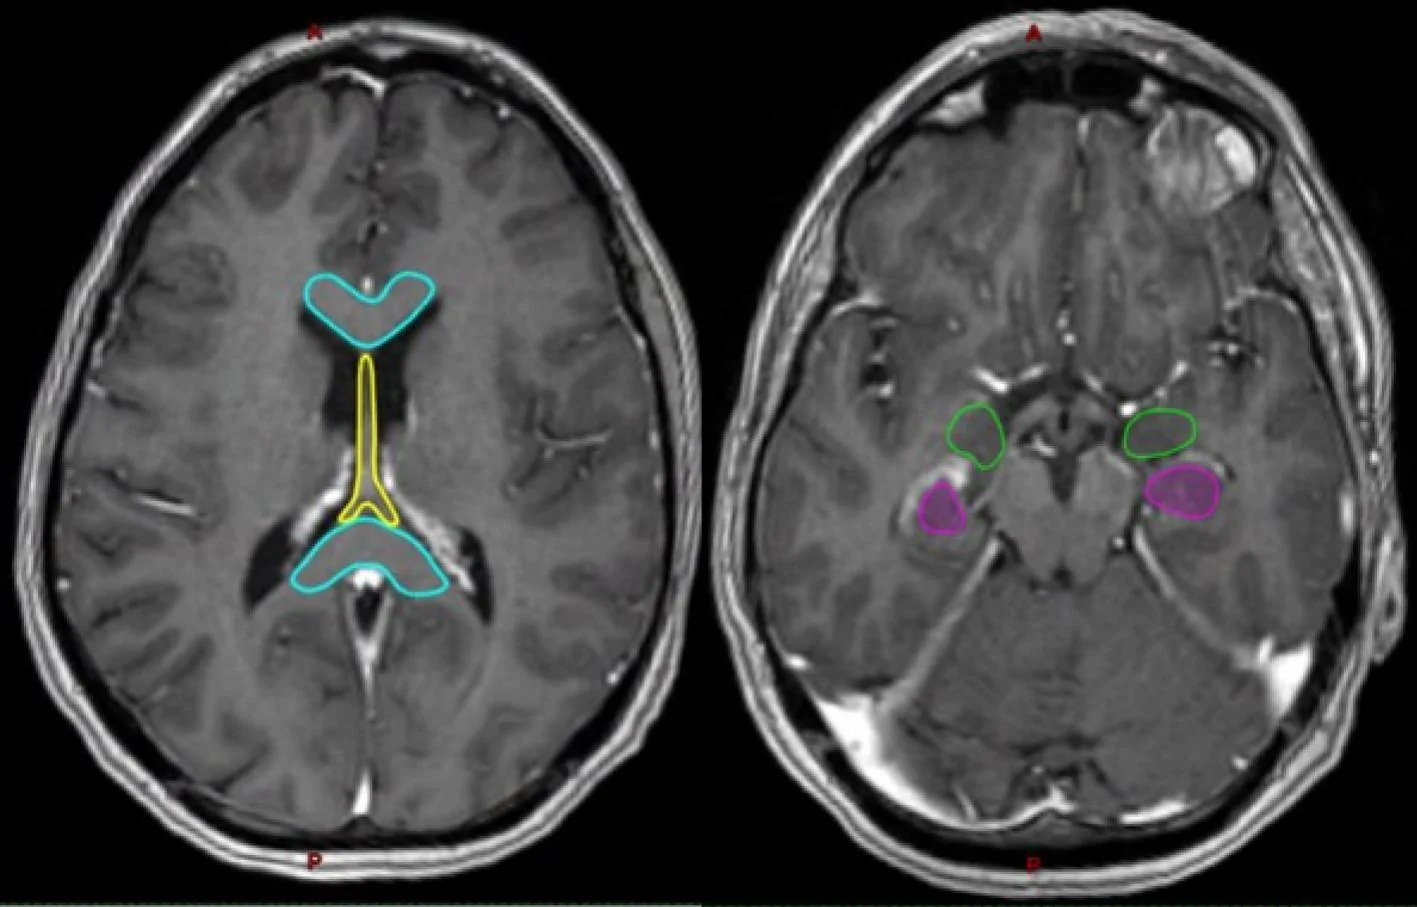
Representative MC contours on MRI brain with contrast which includes corpus callosum (cyan), fornix (yellow), amygdalae (green), and hippocampi (pink). (For interpretation of the references to colour in this figure legend, the reader is referred to the web version of this article.)
